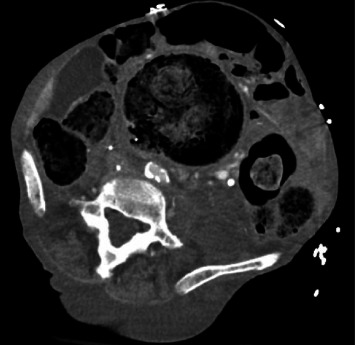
CT abdomen and pelvis axial view. Three filter legs visible, including image right (patient left) where the filter leg is within the colon lumen.
